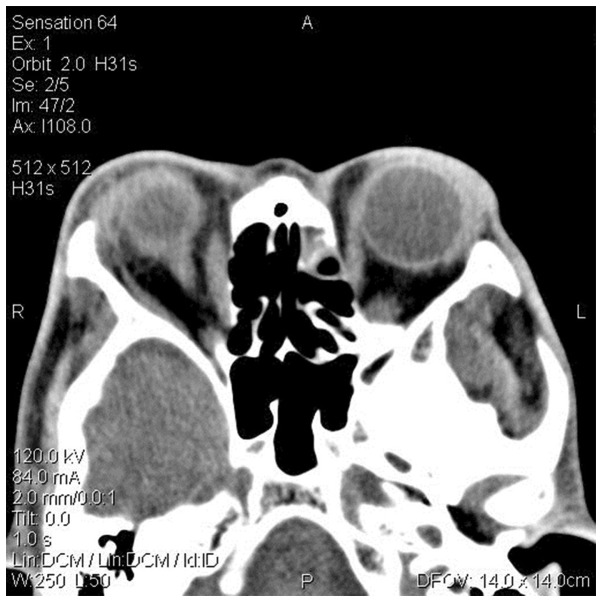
CT scan demonstrating enlargement of the bilateral intraocular rectus.
